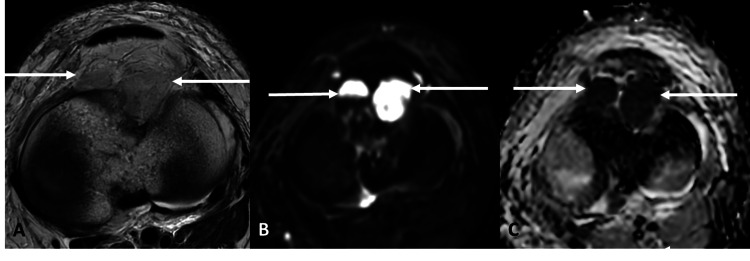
MRI of the left knee joint A, B, & C: Axial sections of the knee joint in T2 (A), DWI (B), and ADC (C) sequences. Two relatively well-defined fluid collections (solid white arrows) show restricted diffusion with corresponding ADC drop noted in the anterior aspect of the knee joint. DWI: diffusion-weighted imaging; ADC: apparent diffusion coefficient
